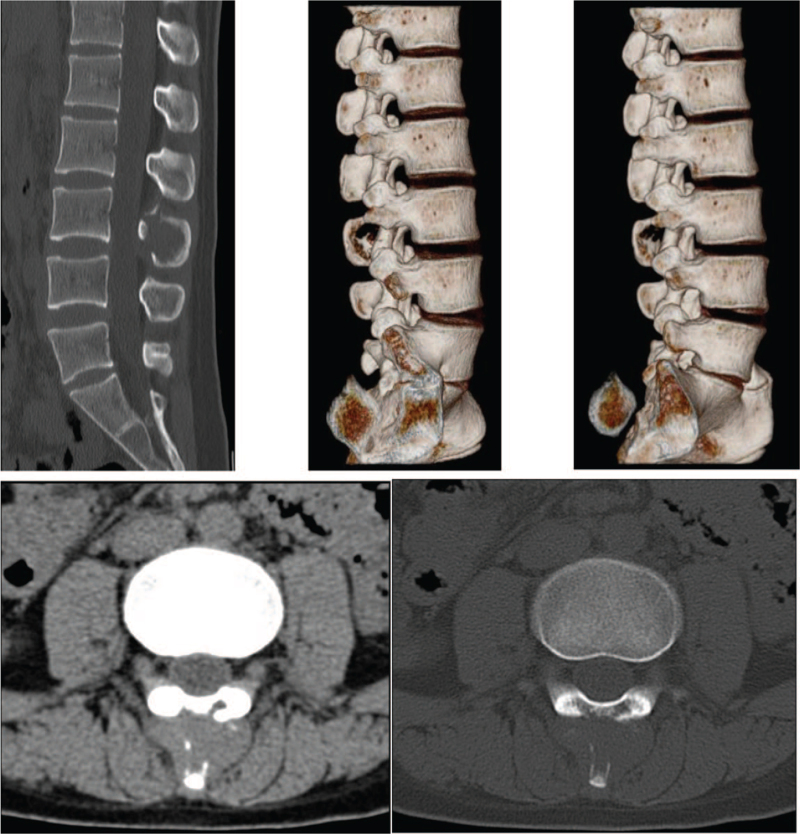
Preoperative CT images. The L3 pedicle and spinous process bone destruction, local bone cortex hours, soft tissue density shadow can be seen, CT value is 51HU, the boundary is unclear, and the range is approximately 1.9 cm × 1.9 cm.
